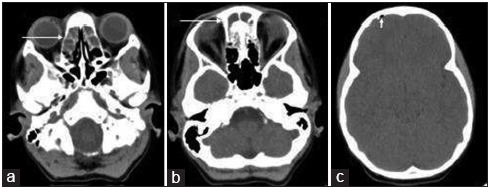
A 9-year-old boy presenting with headache and vertigo found to have frontal sinusitis and pneumocephalus. (a-c) Axial non-contrast CT images of the head demonstrate fluid attenuation within the bilateral ethmoid sinuses, frontal sinuses, and a small right frontal focus of pneumocephalus (arrows).
